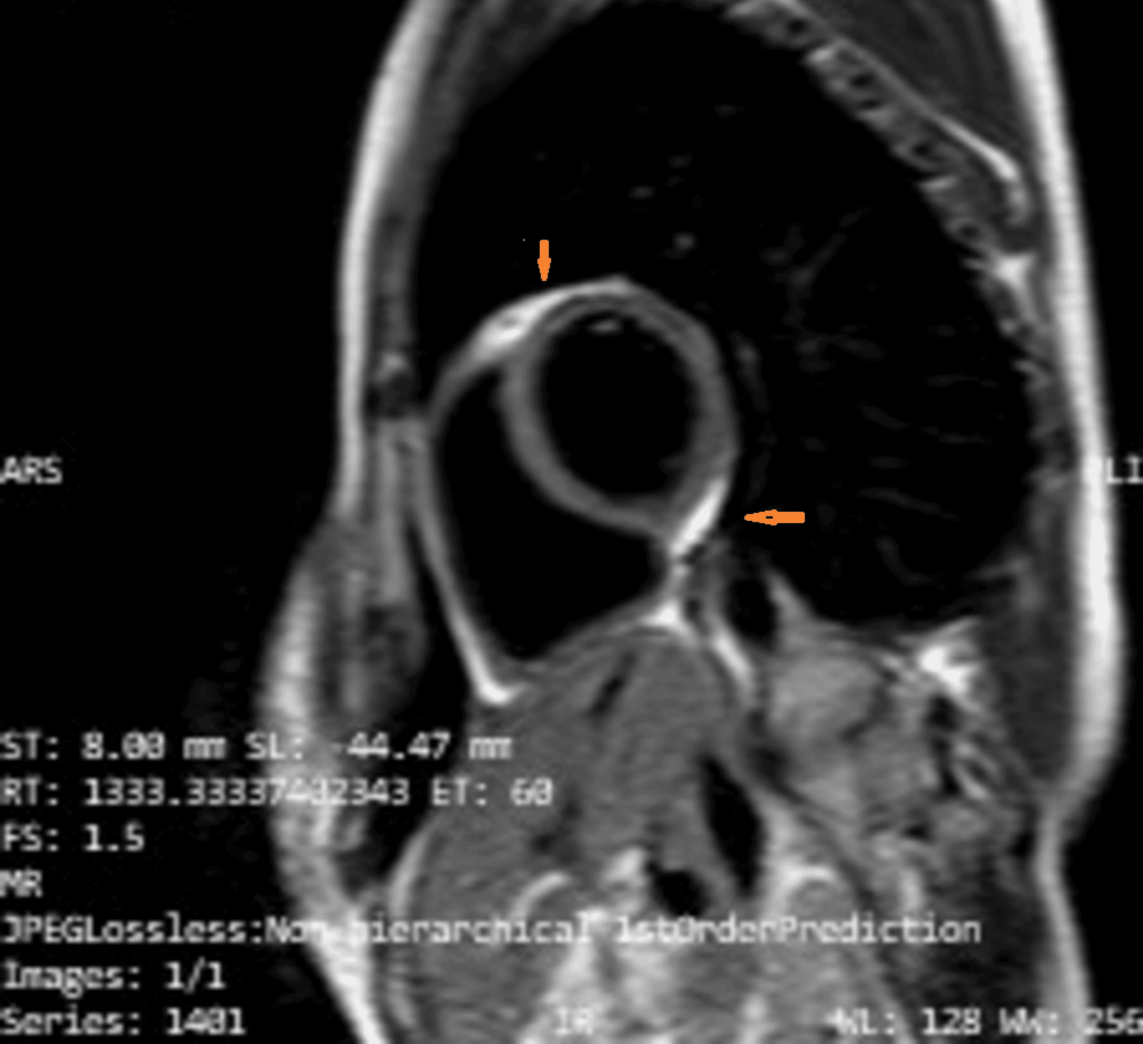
T2-weighted Turbo Spin Echo with Fat Saturation MRI sequence showing involvement of the left ventricular myocardium with basal and mid-cavity antero-inferior myocardial hyperintensity (orange arrows).
